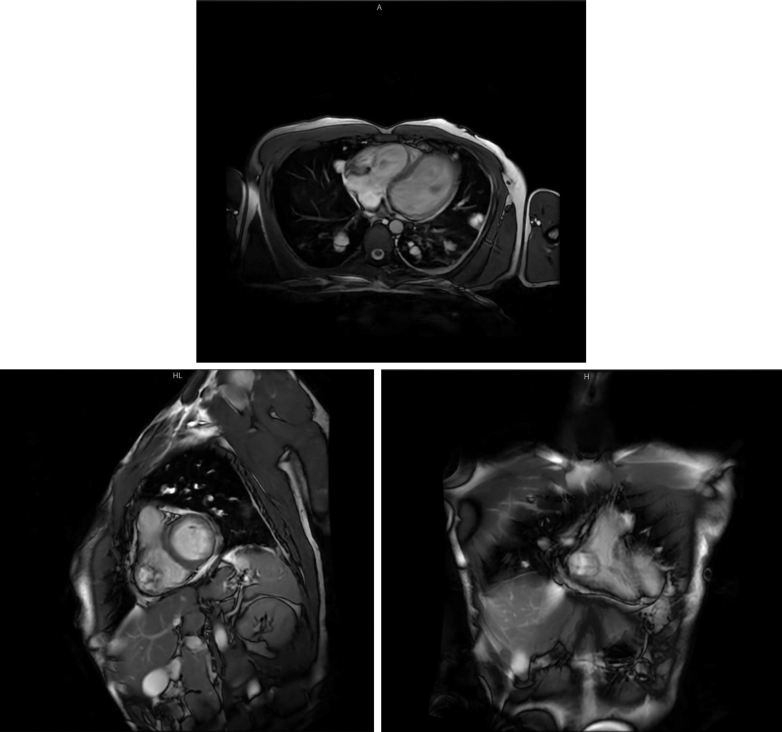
CMR Demonstrating a Benign Multicystic Lesion in the RV (A-C) Cardiac mass revealed a multicystic lesion measuring 26 × 30 mm, attached to the RV free wall. The lesion appeared hyperintense on both T1- and T2-weighted images. There was no evidence of thrombus, no perfusion on first-pass imaging, and no late gadolinium enhancement—features consistent with a benign, avascular lesion. RV function was preserved. The left ventricle demonstrated normal indexed volumes, wall thickness (maximum ∼8 mm at the basal anteroseptum), and ejection fraction. No regional wall motion abnormalities were observed at rest. There was no left ventricular or RV hypertrophy. CMR = cardiac magnetic resonance imaging; RV = right ventricle.
